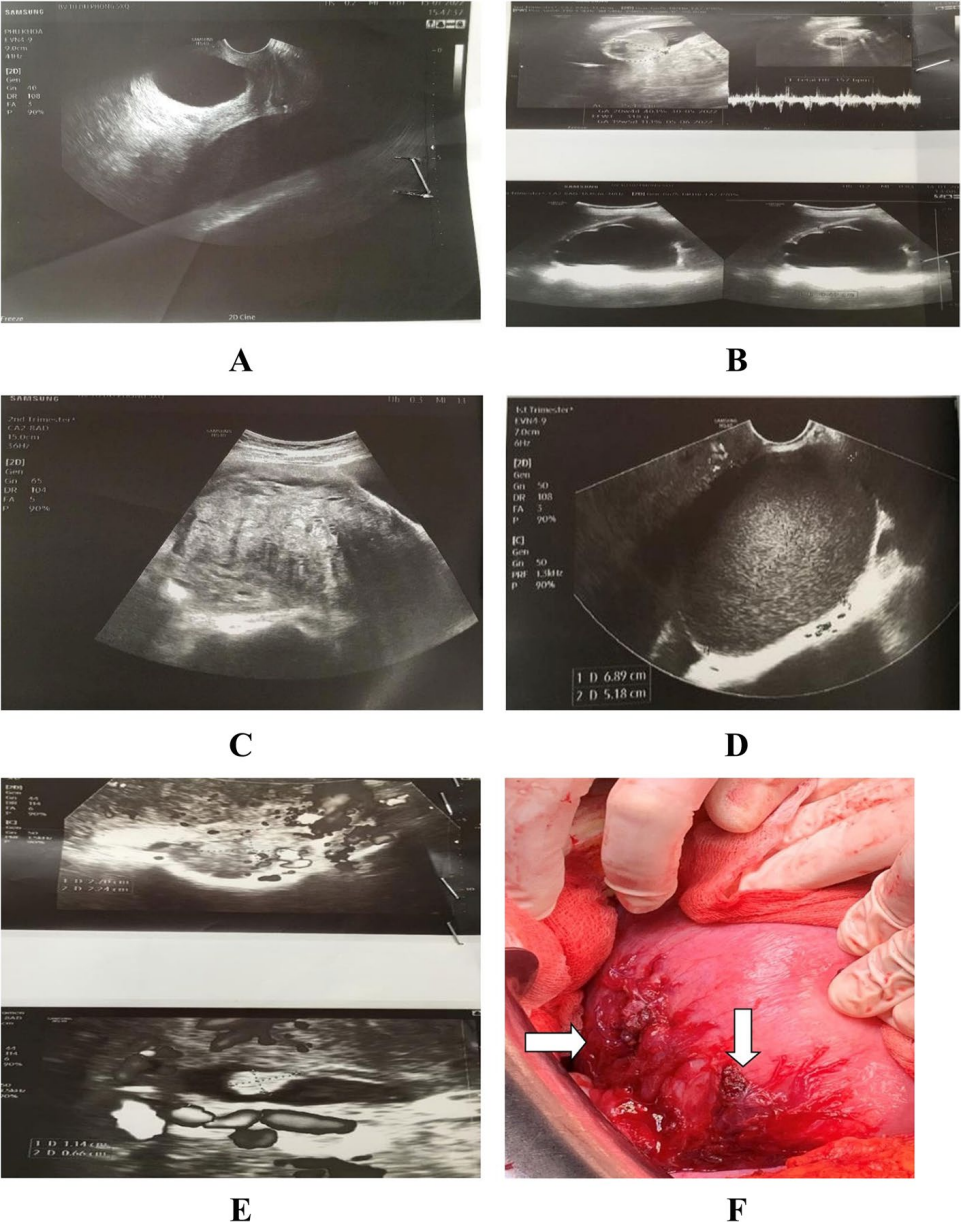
Ultrasound scan shows as follows: A free fluid in the pelvic cavity. B Single alive fetus at 21 weeks and 3 days of gestation and maternal hydronephrosis at the third grade. C Adenomyosis image. D Endometriotic cyst. E Hypervascularity on the lateral wall of the uterus. F Abnormal appearance with laceration on the serosal surface of the uterus and vessel ligations (white arrow) were performed during exploratory laparotomy
